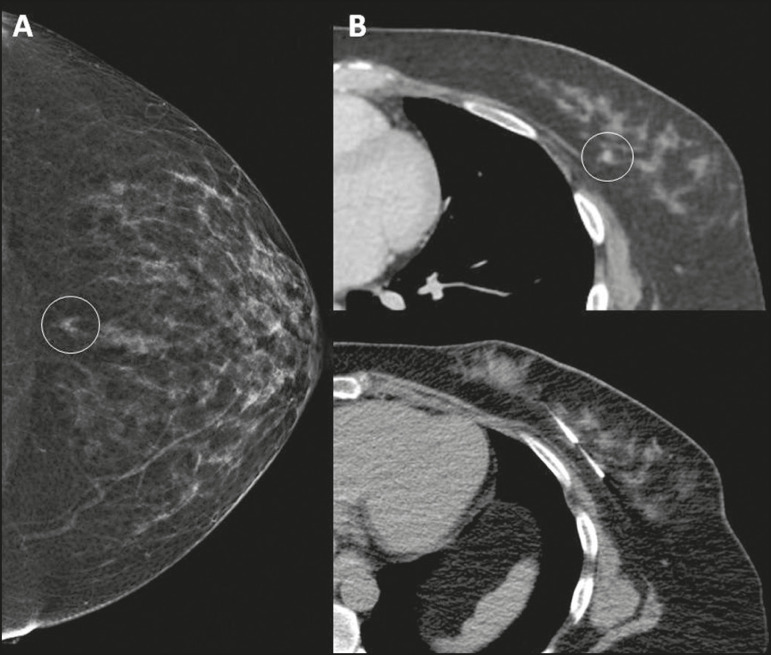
A: Mammography showing focal asymmetry in the posterior depth of the inner quadrants of the left breast (circle). B: CT showing a enhancing mass at the same topography of the mammographic finding (circle) and needle positioned to perform the biopsy.
